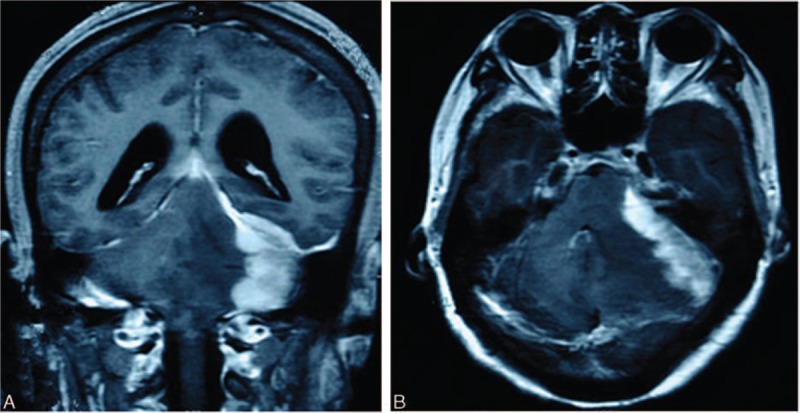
Contrast-enhanced MRI of the brain showed an enhanced mass, measuring 5 cm × 5 cm × 3.5 cm in the right CPA.
